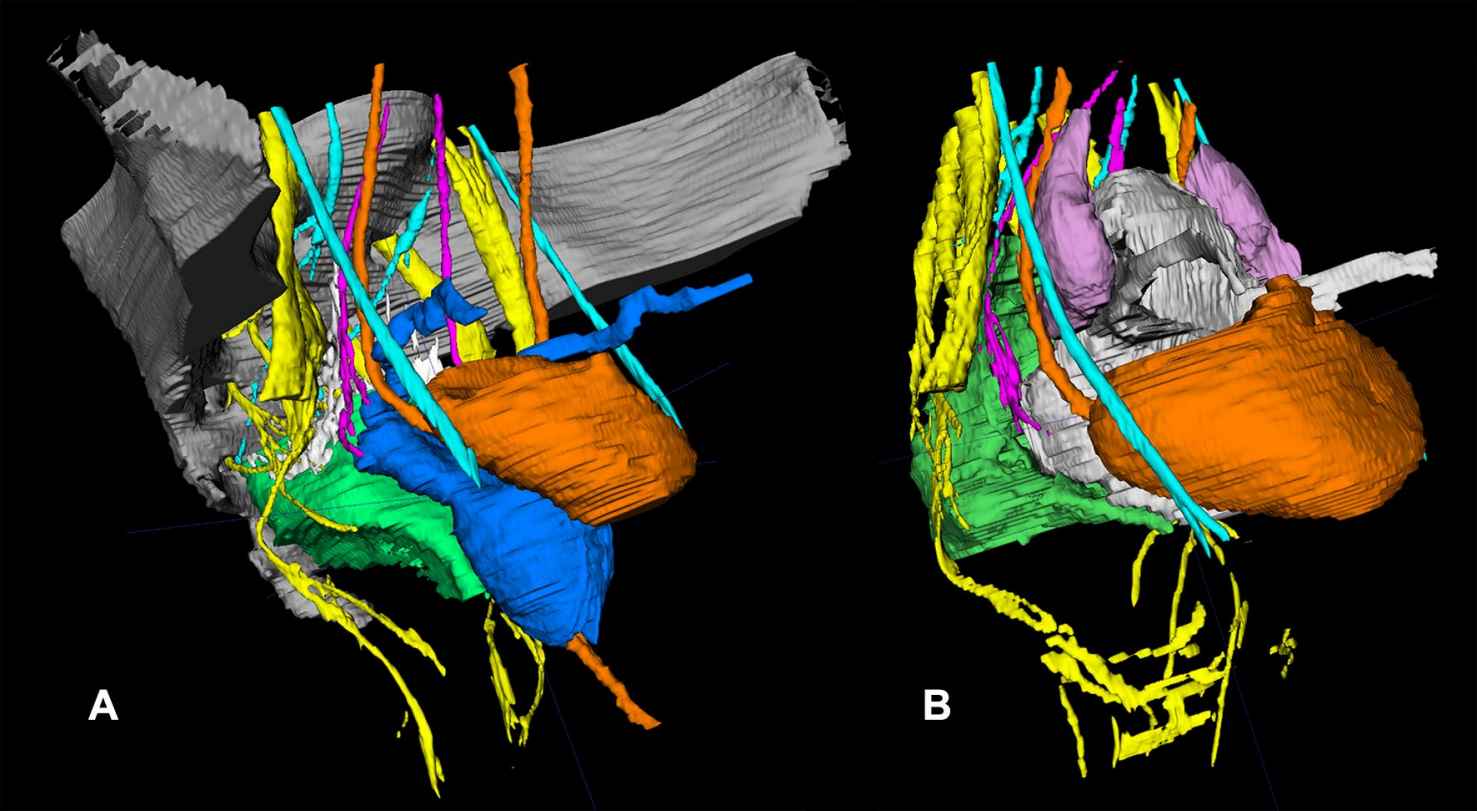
3D MRI pelvic nerve topography showing the anatomical relationships between the pelvic nerves and the pelvic organs in a male (A) and in a female volunteer (B). A video of such a delineation is available as supplementary material 1. The following pelvic organs and nerves can be distinguished: bladder/ureter and urethra (orange), mesorectum of the low and partially mid-rectum (green), prostate/seminal vesicles/deferent duct (in A, blue), sacrum and partially iliac bone (in A, gray), uterus/vagina/round ligament (in B, gray), ovaria (purple), SNs/lumbosacral plexus/PN/LAN (yellow), ST (turquoise, running ventrally along the sacrum), ON (turquoise, the most ventrally situated nerve), SHP/HN (pink), IHP (in A, white/distal side pink; in B the pink HN is running through in the IHP). (Color figure online)
